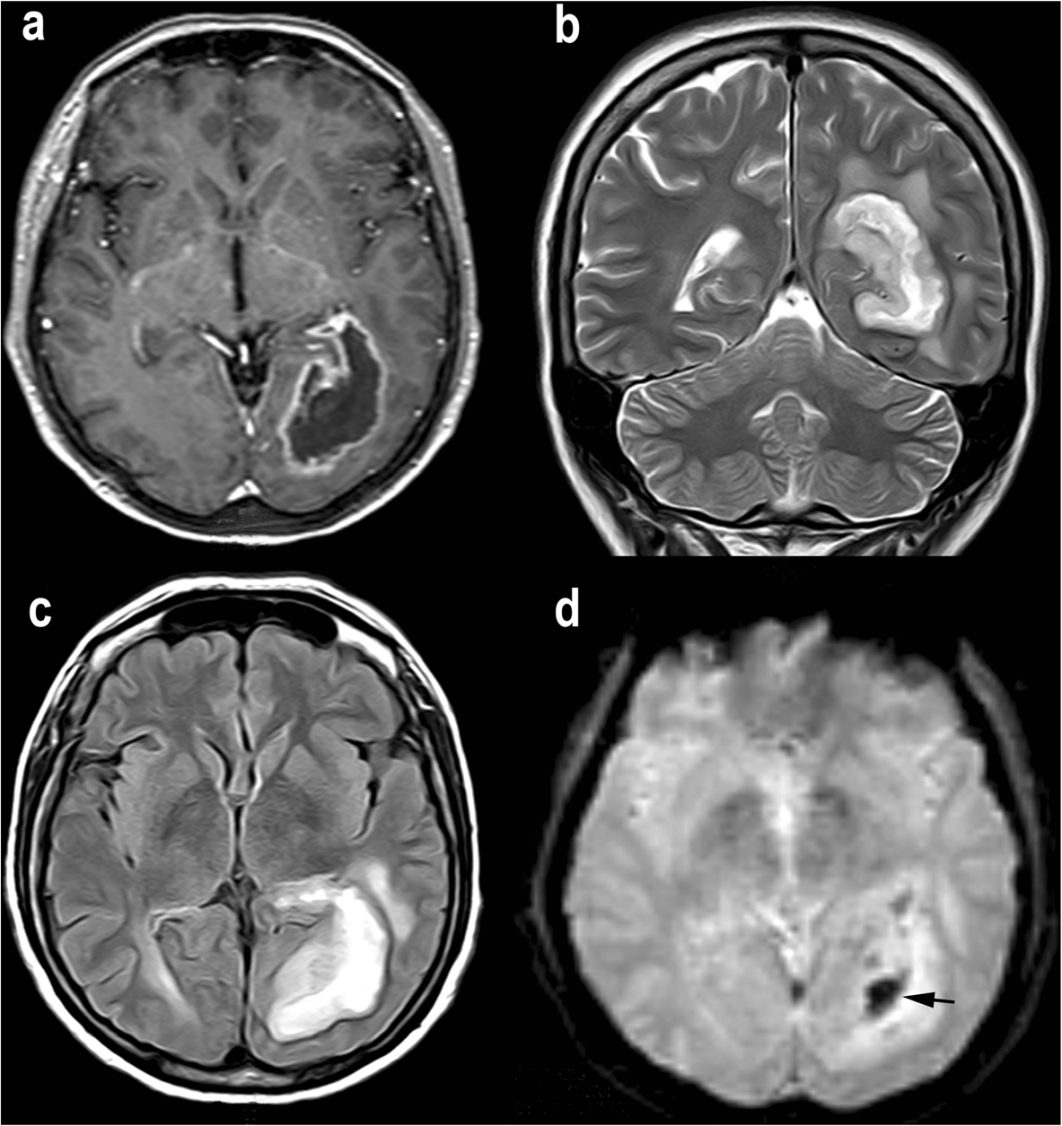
Example of MR images of glioblastoma (grade IV). a-c, Signs of necrosis, identified as regions within the neoplasm with hypointensity on contrast-enhanced axial T1-weighted images (a) and hyperintensity on T2-weighted images (b) and FLAIR images (c). d, Signs of hemorrhage seen as an area of hypointensity (arrow) due the paramagnetic blood breakdown products on the axial EPI T2*-weighted images.
